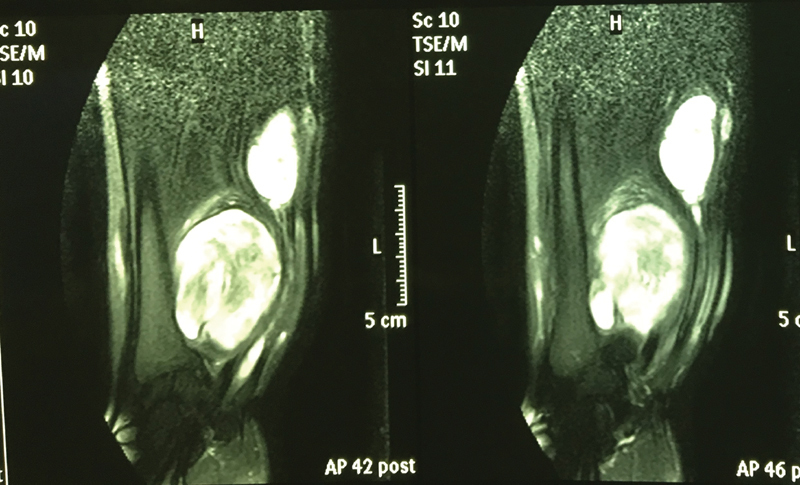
Magnetic resonance imaging (MRI) examination indicated two soft tissue tumors located by the ulnar nerve.
